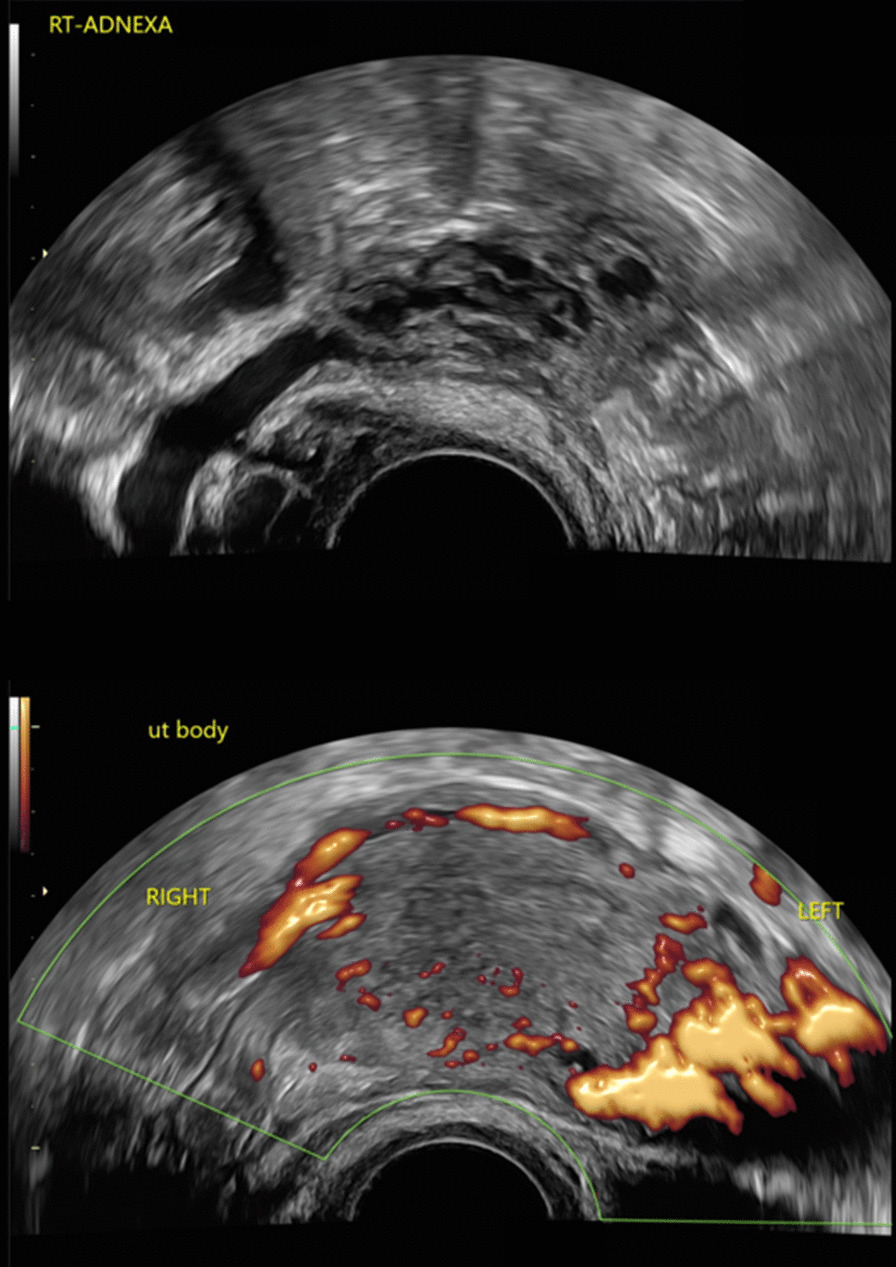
Transvaginal ultrasound images: Colour flow mapping demonstrated tortuous dilated vasculatures over the cervix, appearing more prominent over the paracervical region
